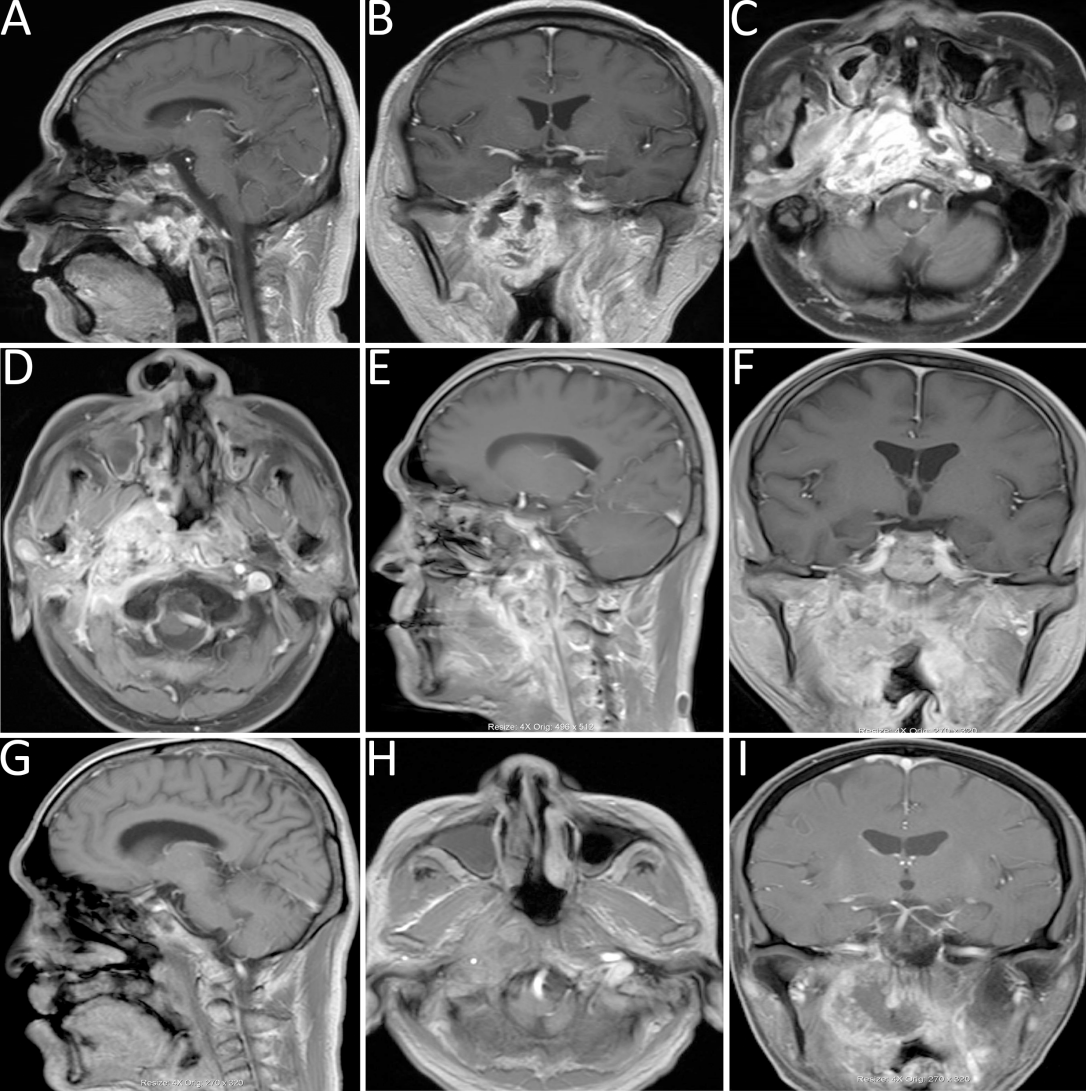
(A-C) Head MRI demonstrated abnormal signal intensities in the nasopharyngeal roof and right parapharyngeal space, with heterogeneous contrast enhancement and linear enhancement at the margin of the left nasopharyngeal wall. (D-F) MRI revealed a space-occupying lesion in the right parapharyngeal space. (G-I) Follow-up MRI indicated marked liquefaction and necrosis within the surgical cavity, reduction of the primary lesion, and findings consistent with a partial response (PR).
